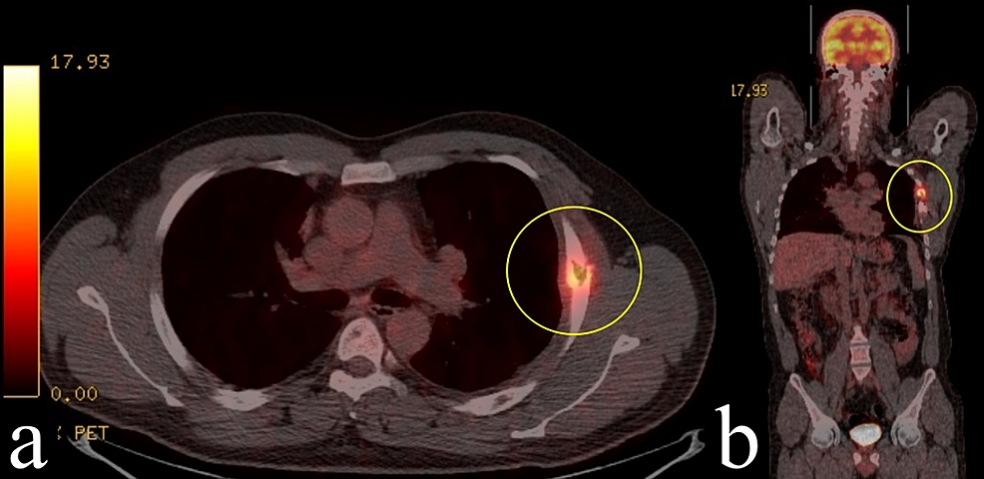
Osteolytic lesion on the left fourth rib (yellow circle) with pathologic uptake (SUVmax: 6,5) on PET/CT axial (a) and coronal (b) images The second admission was in 2021. SUVmax, Maximum standardized uptake value; PET/CT, positron emission tomography/computed tomography.
